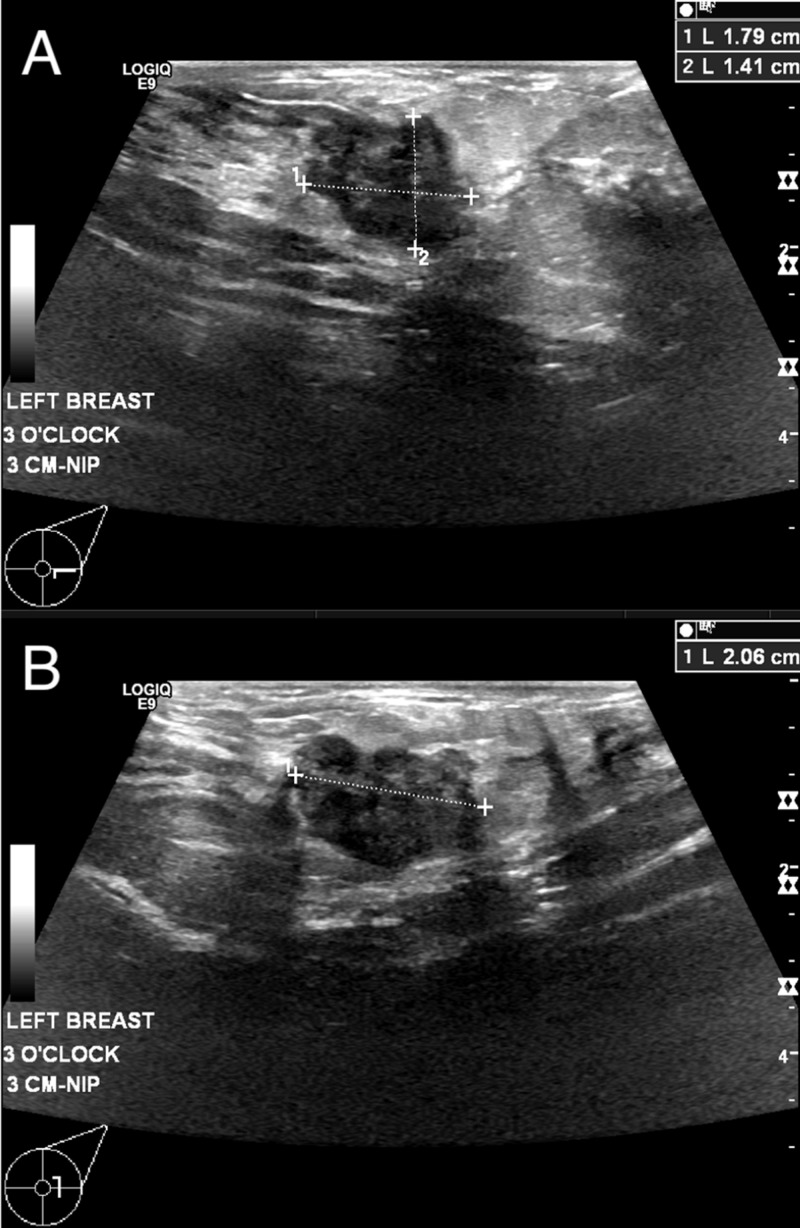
Ultrasound of the Palpable Mass An ultrasound of the left breast demonstrates overlying clinically palpable disease with the transducer in the transverse (A) and sagittal positions (B). Precise measurements can be observed in the upper right panes of the corresponding images.
